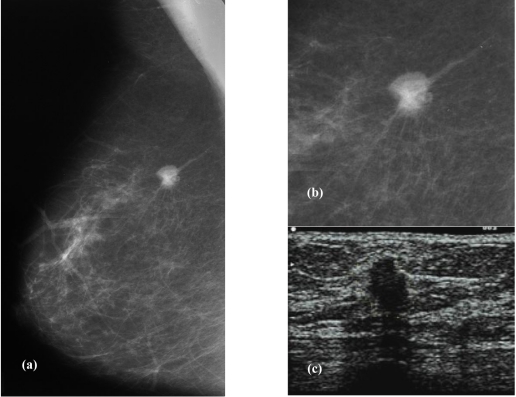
(a) A nodule seen on the right MLO view (arrow). (b) Magnification showed the same nodule to have a spiculated margin suspicious of malignancy. (c) Ultrasound confirms the presence of an invasive ductal carcinoma (biopsy proven) showing a hypoechoiec nodule with a ‘taller than wide’ appearance.
